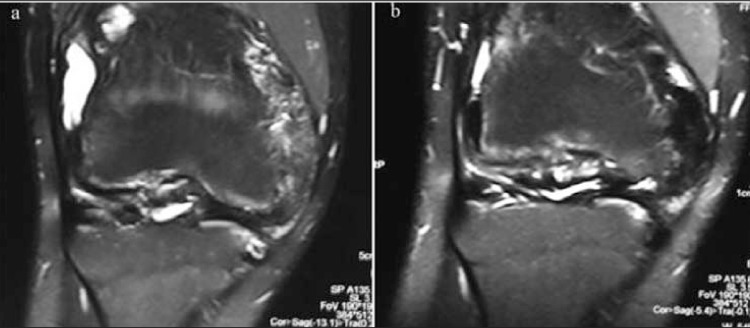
The coronal fat-saturated T2 weighted MRI images beforeradiosynovectomy show narrowing in joint space, synovial hypertrophy,moderate amount of effusion (a) and a decrease in the amount of effusion,subchondral hypointense areas and cartilage defects in the lateral sideand development of hypointensity due to hemosiderin pigment accumulationin the hypertrophic synovium at the medial side is observed in theimages obtained 6 months after radiosynovectomy (b)
